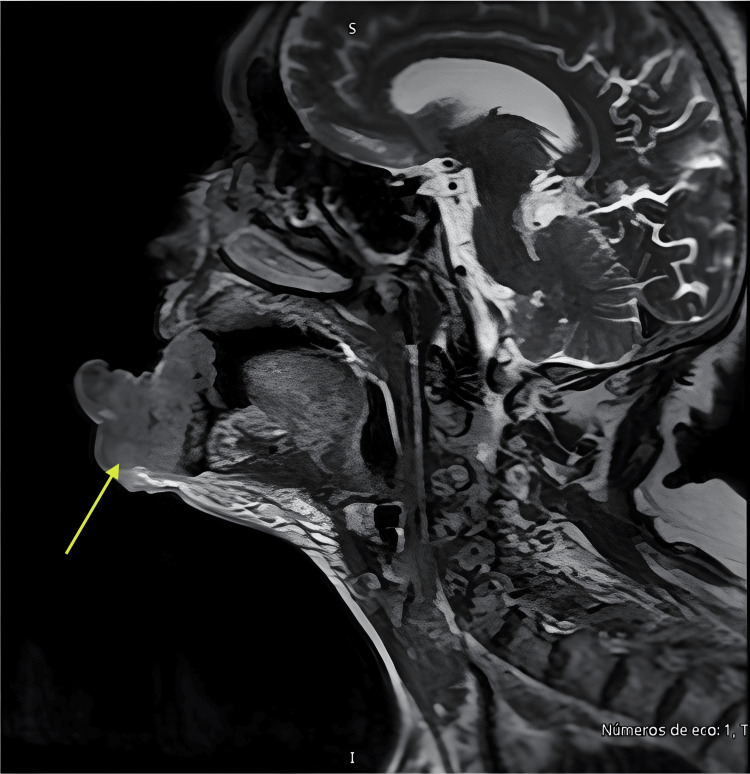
Sagittal MRI demonstrating locally advanced mandibular tumor with deep soft tissue extension Sagittal MRI demonstrates a large infiltrative lesion arising from the mandibular alveolar ridge, with extension into adjacent deep soft tissues of the floor of the mouth and submandibular region. The lesion exhibits heterogeneous signal intensity and mass effect, with distortion of normal anatomical planes (yellow arrow).
